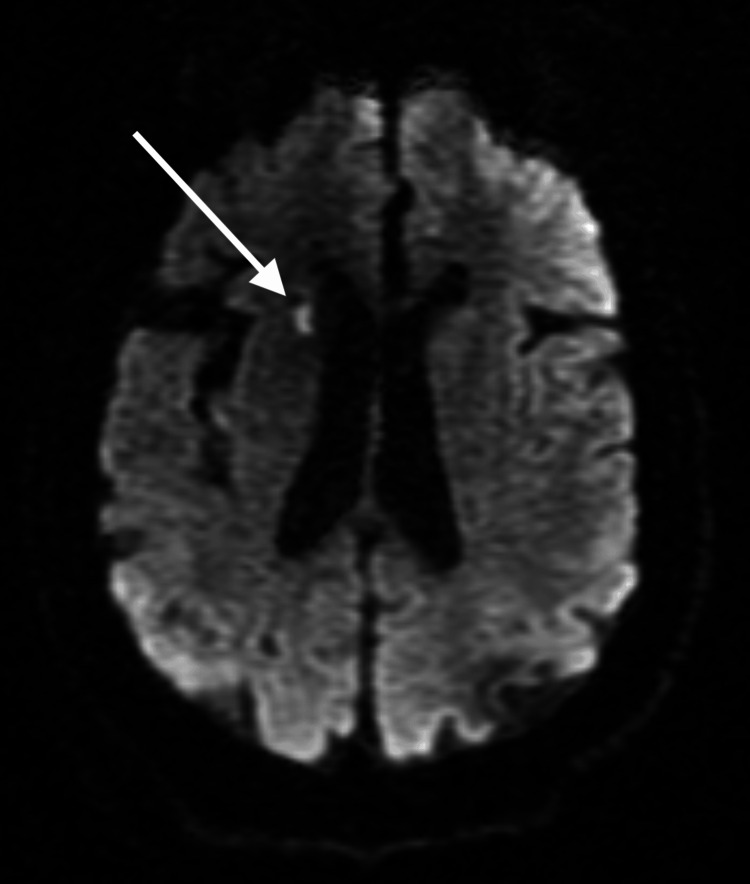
Diffusion-weighted T2 MRI showing infarct The arrow in this MRI scan points to an acute to subacute lacunar infarction in the right head of the caudate nucleus. The radiologist noted no acute processes that could explain the patient's encephalopathy.
